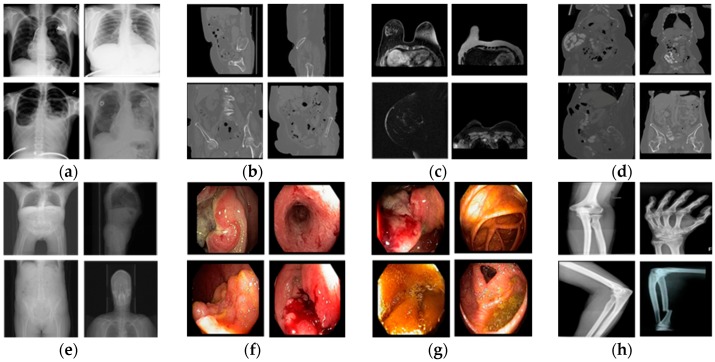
Selected example images for showing high intra-class variance; (a) chest X-ray in Figure 5b-1; (b) urography CT in Figure 5b-2; (c) breast MRI in Figure 5b-3; (d) uterus CT in Figure 5b-5; (e) CT topogram in Figure 5c-3; (f) rectum in Figure 5d-1; (g) colon in Figure 5d-2; (h) bones X-rays in Figure 5e-6.
